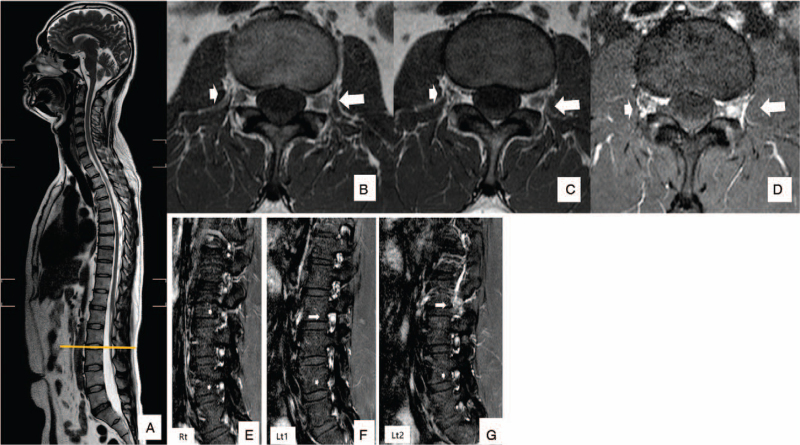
A–D, Sagittal MRI shows no definite evidence of clinical radiculopathy of the lumbar spine (A). Axial images of L2–3. Two consecutive serial axial images of L2–3 show asymmetrical thickening, or swelling of the Lt dorsal root ganglion (DRG, long arrow), compared with the contralateral DRG (short arrow) (B, C). Gadolinium enhancement revealed increased contrast enhancement of the involved (Lt) DRG (D). Sagittal image of L2–3 Lt root shows increased enhancement (long arrow, Lt1, Lt2) comparison with nerve roots of the unaffected contralateral side (E), or adjacent segments (F, G) (short arrow).
